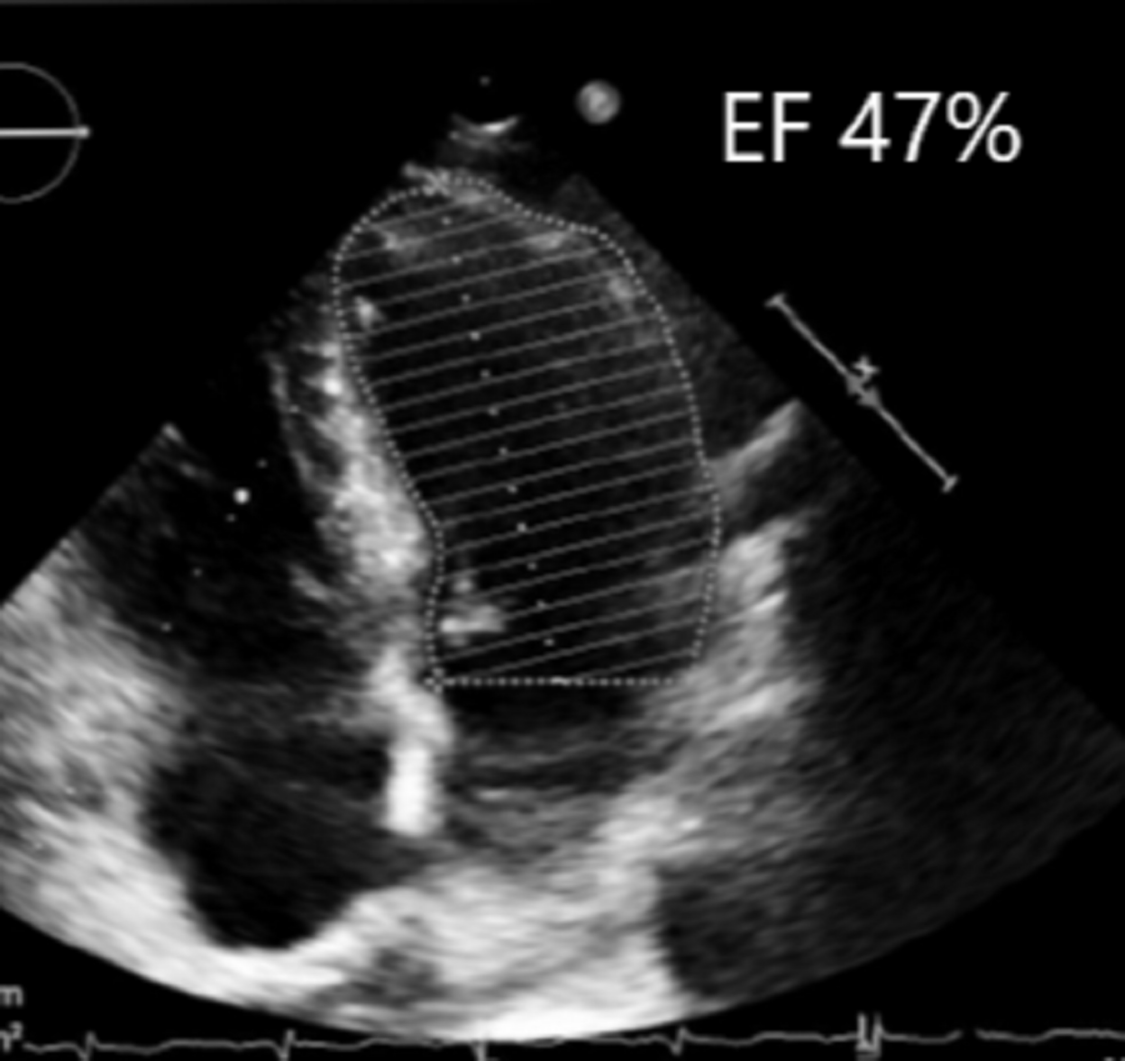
Two-dimensional transthoracal echocardiography showing left ventricular ejection fraction of 47%
